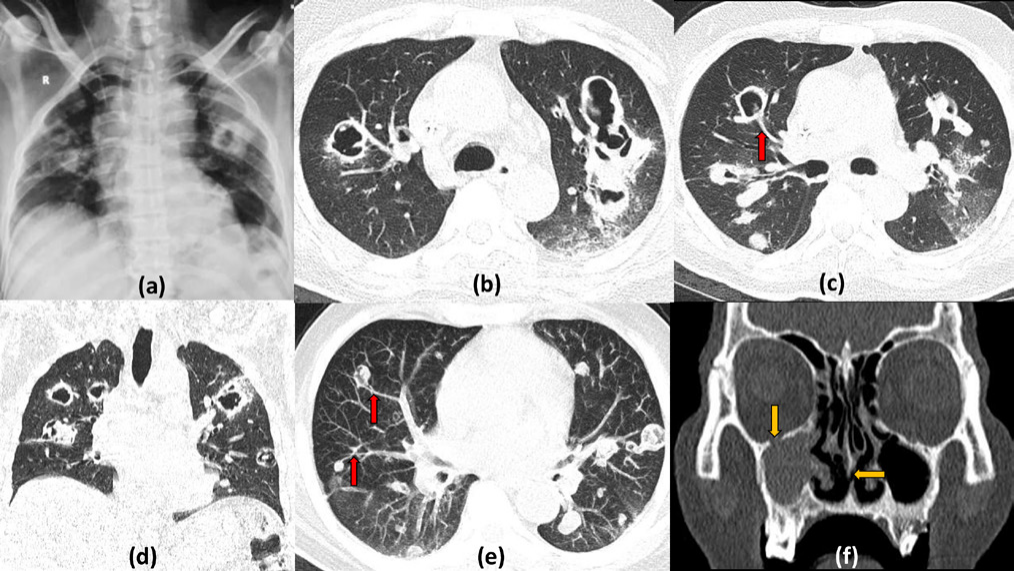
Multiple cavitating nodules of granulomatosis polyangiitis with COVID19 Pneumonitis: 55-year-old male presented to the emergency with acute shortness of breath and fever and tested positive for COVID19. Frontal radiograph shows evidence of multiple variable sized cavitating nodules in the bilateral lung fields. Chest CT lung window sections (b–e) show multiple well-defined nodules of varying sizes, most of which show central cavitation with *feeding vessel sign* seen (red arrows) better on the Maximum Intensity Projection image (e). Few focal ground glassing is seen in the posterior and basal areas of bilateral lung fields. Coronal CT of the paranasal sinuses, bone window (f) shows opacification of the right maxillary sinus with focal destruction of the nasal septum and erosion of the right orbital floor (yellow arrows).
